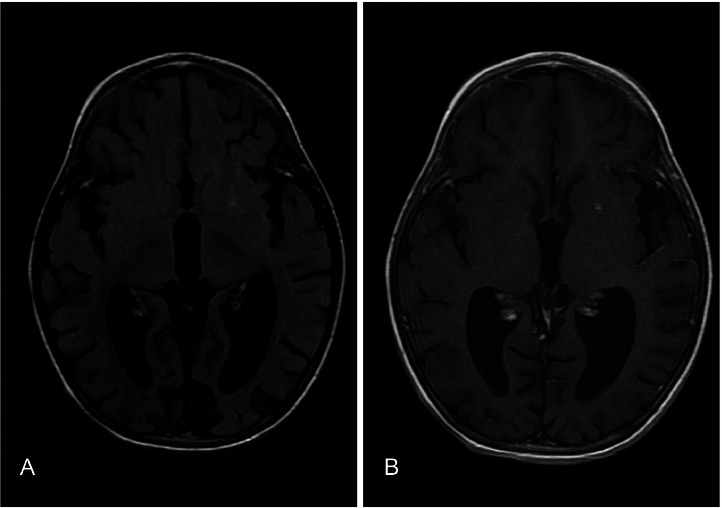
T2 FLAIR (A) and 3D T1 SPGR PC (B) sequence showing a tiny hyperintense focus showing punctate post contrast enhancement in the left putamen, without any perifocal edema.
